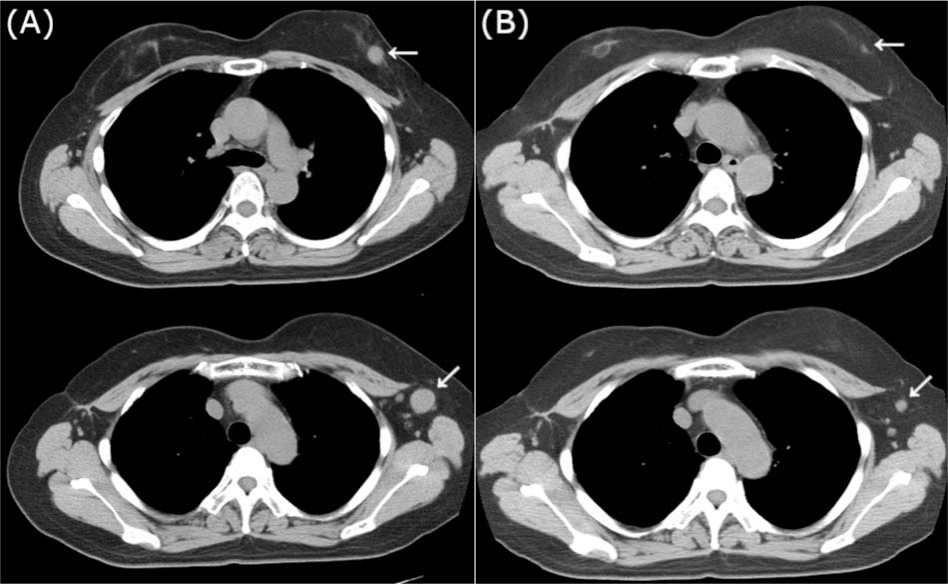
CT images of left breast tumor before and after treatment. (A) Chest CT displaying a nodule in the left breast (top, arrowhead) and lymph node swelling in the left axilla (bottom, arrowhead); (B) Tumors in both the left breast (top, arrowhead, arrowhead) and the axillary lymph node (bottom, arrowhead, arrowhead) shrank after carboplatin and paclitaxel administration.
